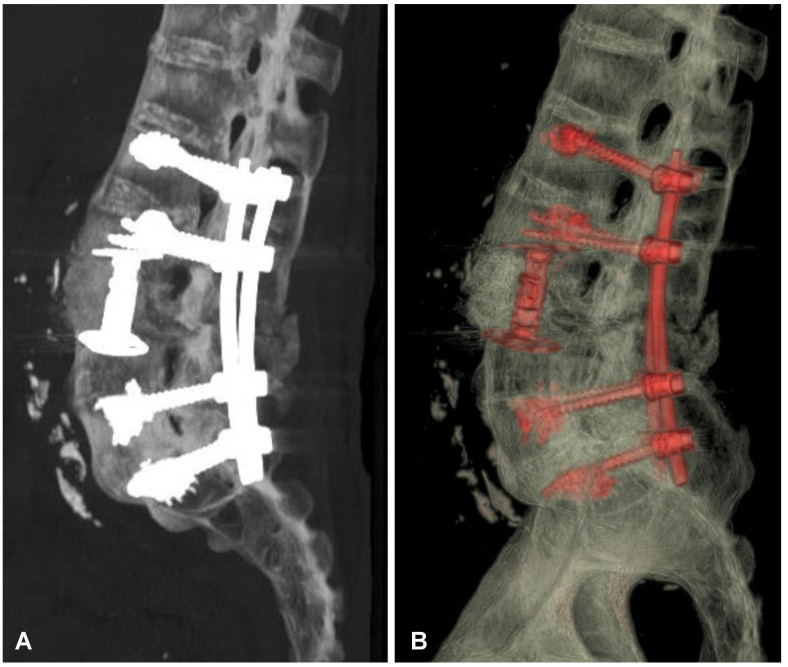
Postoperative CT-based reconstruction of the lumbar spine demonstrating correct positioning of the anterior and posterior spinal implants at the L2-L3-L5-S1 levels (A,B).
